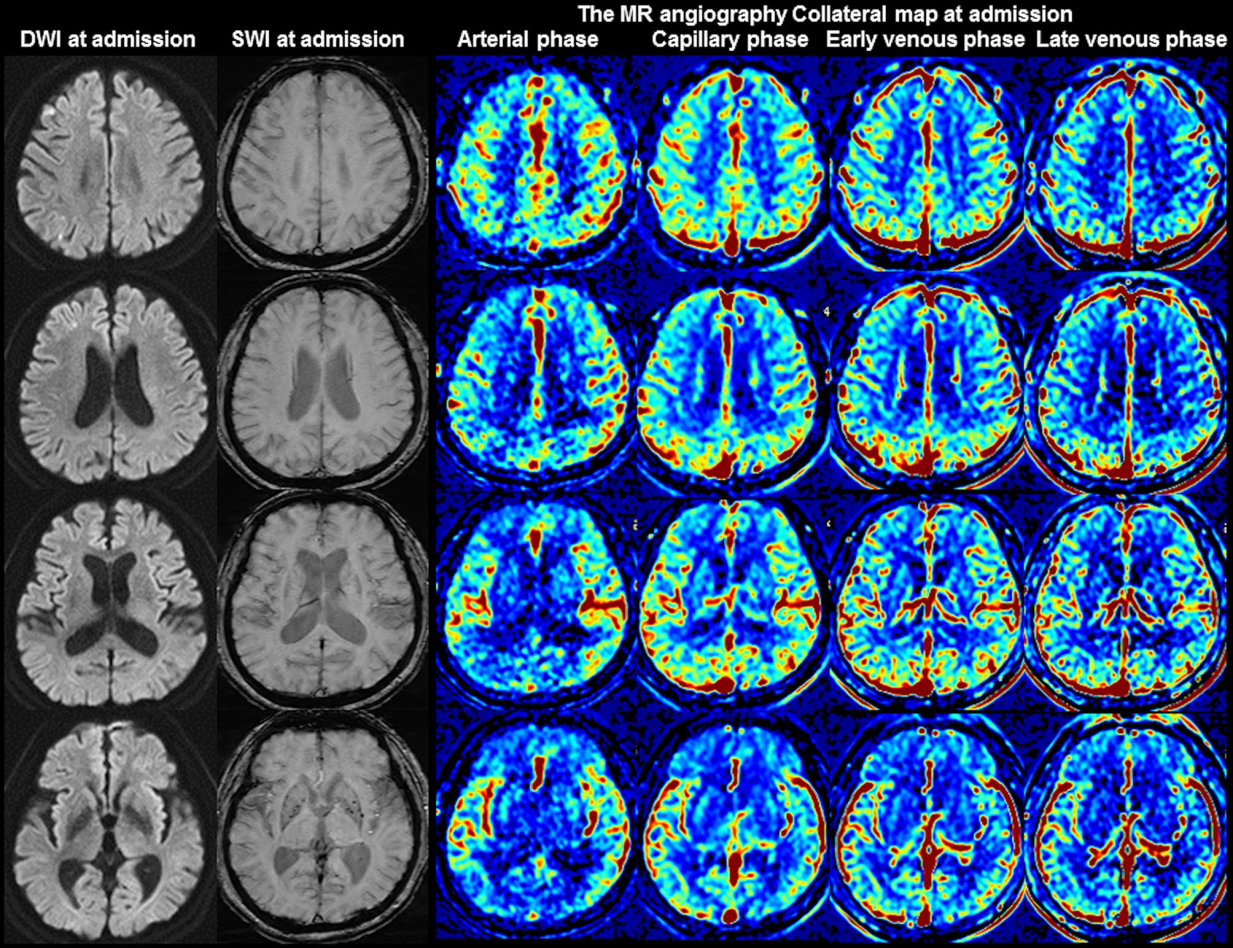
Images of a 68-year-old man with greater than 70% stenosis of the right proximal internal carotid artery. The premorbid modified Rankin scale (mRS) score of this patient was 0, and the National Institutes of Health Stroke Scale score at admission was 1. The diffusion-weighted imaging (DWI), susceptibility-weighted imaging (SWI), and multiphase MR angiography collateral map at 44 min after symptom onset are shown. DWI shows acute infarct signals along the border zones of the right cerebral hemisphere, and SWI shows no prominent cortical and medullary veins in the right cerebral hemisphere, representing good collateral status. The MR angiography collateral map shows no collateral-perfusion delay in the right cerebral hemisphere in the capillary phase (MR acute ischemic stroke collateral score of 5: excellent collateral perfusion defined as no or small collateral-perfusion delay in the ischemic MCA territory in the capillary phase regardless of the collateral status in the arterial phase). The patient underwent conservative treatment and recovered, as shown by the 90-day mRS score of 0.
